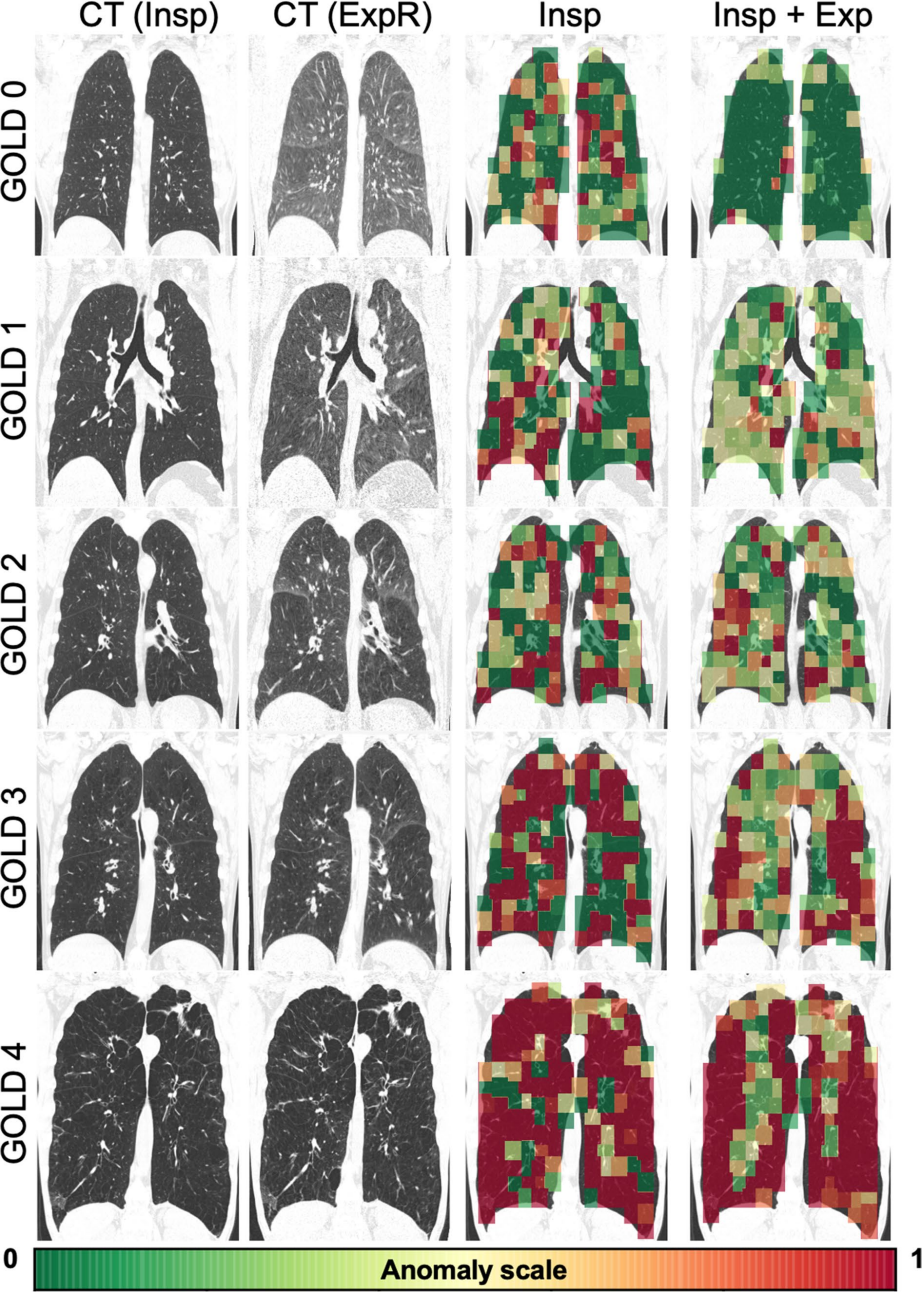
Representative coronal views of the cOOpD score map using the inspiratory image alone (3rd column) and using the Insp and the ExpR image (4th column) on five subjects from COPDGene with different degrees of severity, for a 20% patch-overlapping strategy. CT images are scaled from − 1300 to 50 HU for visualization purposes. Color maps show the normalized patch anomaly score (negative log likelihood), normalized by the min–max normalization corresponding to the 5th and 95th percentiles of the dataset. Red symbolizes higher degrees of severity
